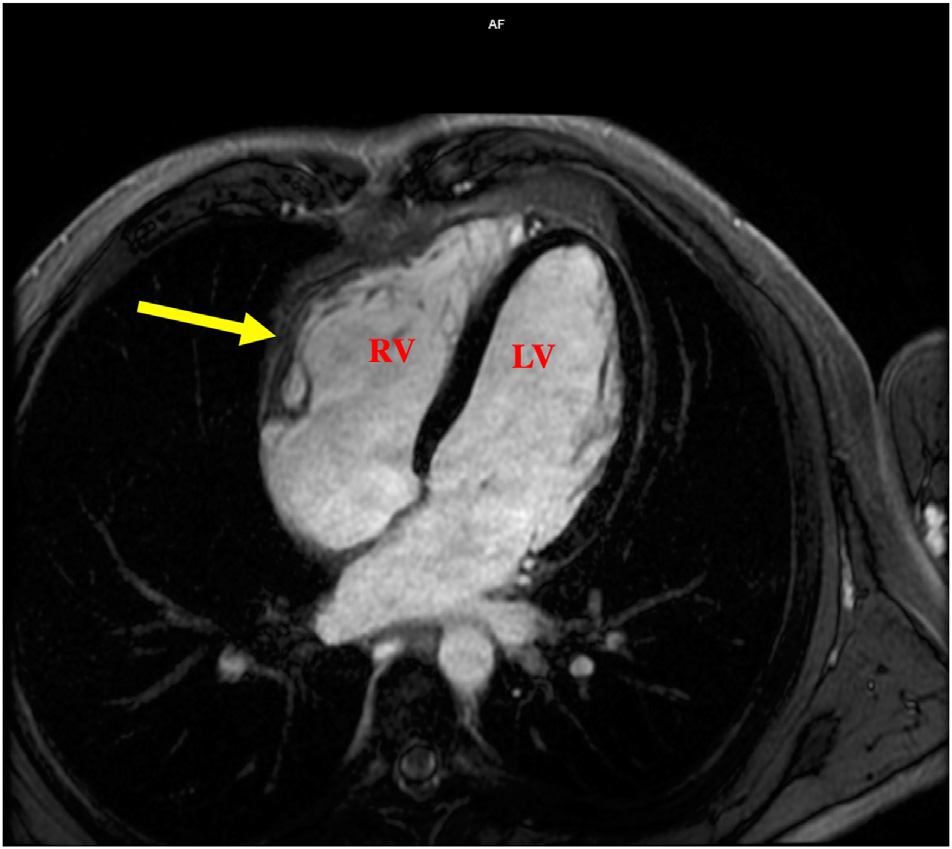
Enlarged right ventricle on cardiac magnetic resonance imaging. The yellow arrow points to the region of focal hypokinesis and mild aneurysm of the right ventricular basal to mid free wall.
